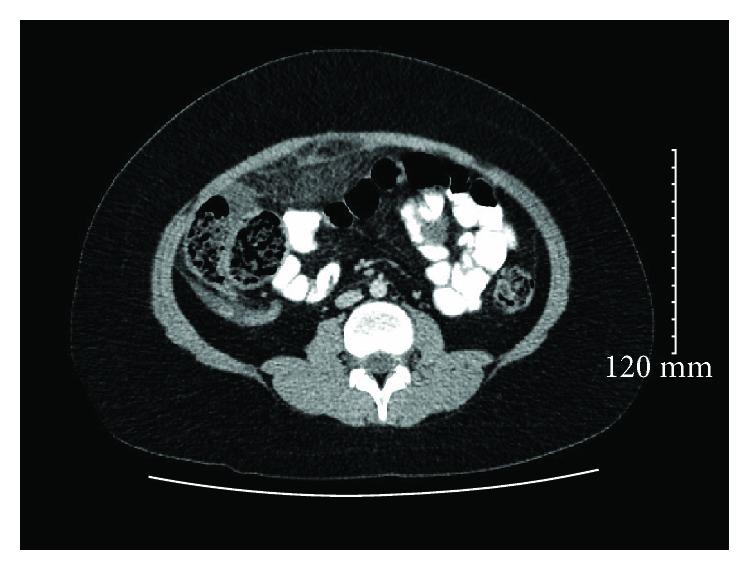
Axial CT demonstrating a small amount of free fluid around the omentum.
